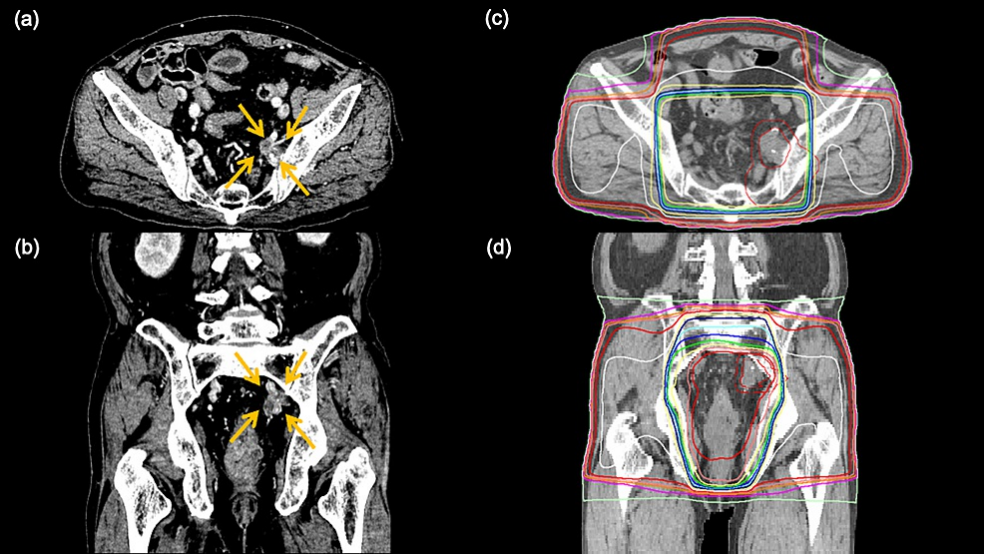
a 71-year-old man with locally advanced rectal cancer (patient 8) The patient undergoes semi-radical radiotherapy encompassing the primary tumor and metastatic pelvic lymph nodes. (a) and (b): axial and coronal views of the tumor (arrows) on CT before irradiation. One of the metastatic lymph nodes is in contact with the left ischiadic nerve (allows). (c) and (d): radiation dose distribution with 95% isodose lines shown in green.
